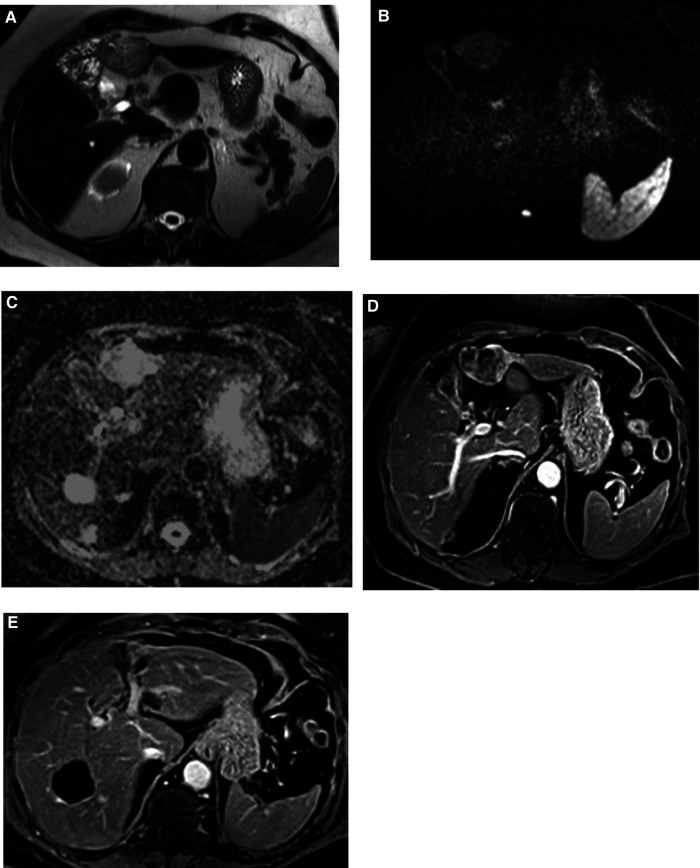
MRI images. Focal oval lesion straddling segments III and IVb showing sharp contours, heterogeneously high signal in T2 (A) and in diffusion-weighted imaging (B) corresponding to heterogeneous hypersignal on ADC map (C). Irregular and globally hypoenhancing behavior on T1 portal venous phase (D). (E) The lesion was located inferiorly to segmental left portal bifurcation, displaying a contact with the segmental branch for segment III without any major distortion or infiltration.
